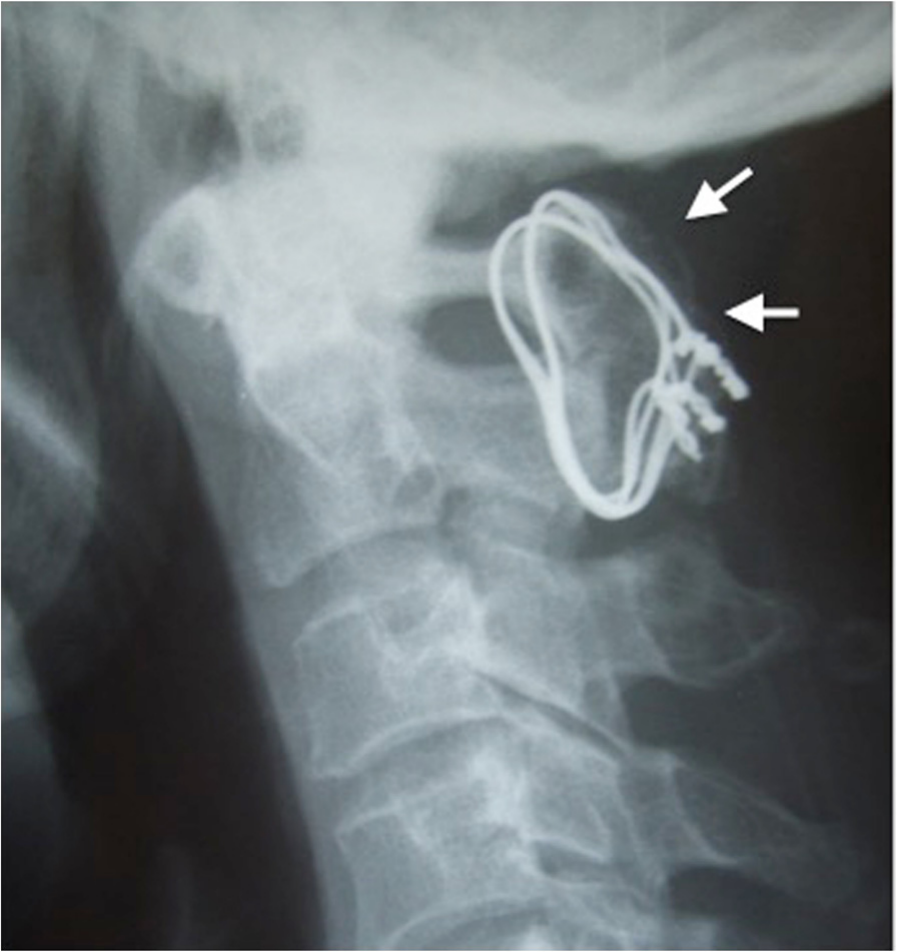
Plain radiography 7 years after operation. Plain lateral radiography shows complete bone union (*arrows*)
